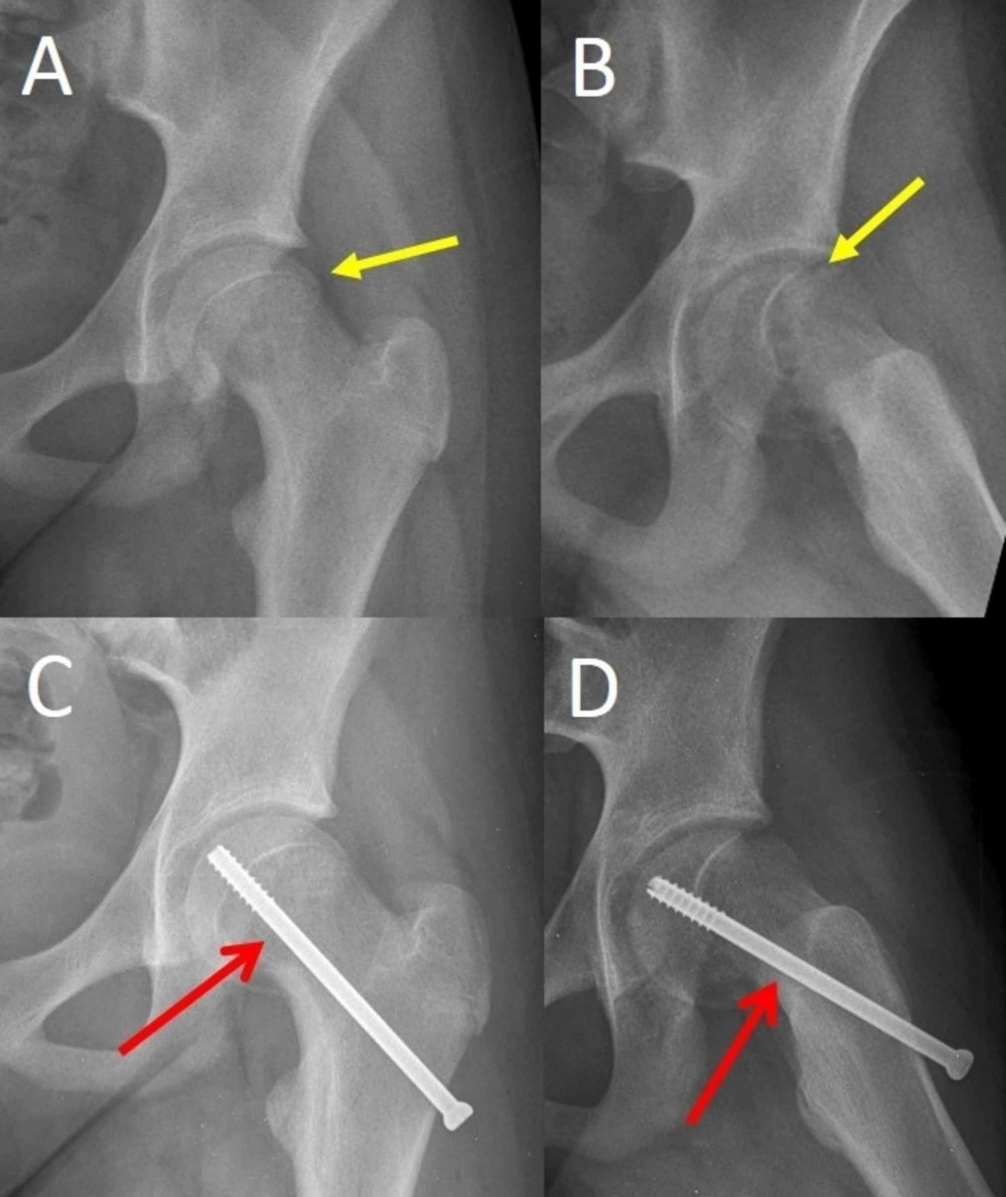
Stable SCFE of the left hip of a 13-year-old girl and in situ stabilization with one cannulated screw (A,B) Anteroposterior and frog-lateral pelvis x-ray scans indicate the slip (yellow arrows). (C,D) Anteroposterior and frog-lateral views of the pelvis indicate in situ stabilization with one cannulated screw (red arrows). SCFE: slipped capital femoral epiphysis
